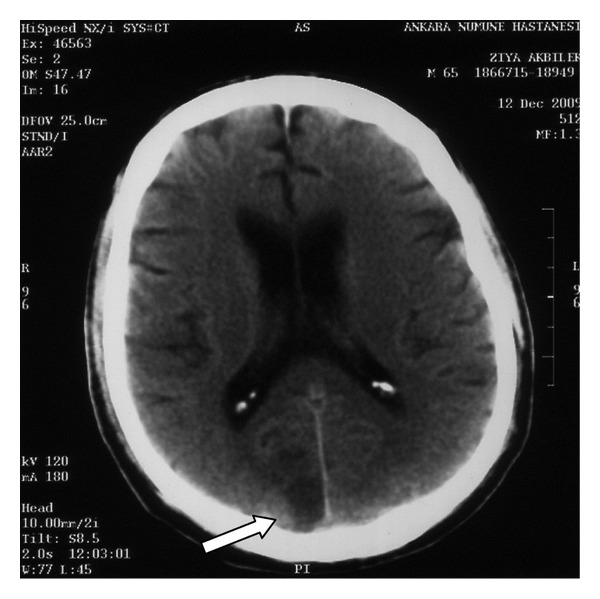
Right occipital infarct area in the CT scan (white arrow).
